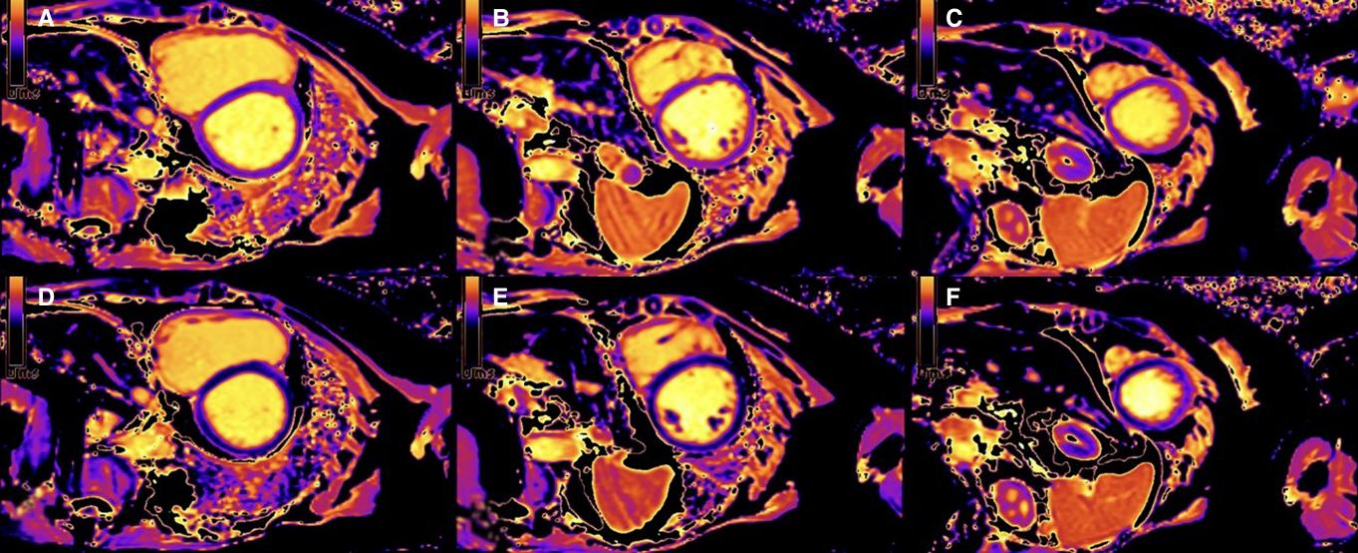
Cardiac magnetic resonance imaging: sequence of T1 maps, short-axis images. (*A–C*) in diastole with values of relaxation times (tables), (*D–F*) in systole.
